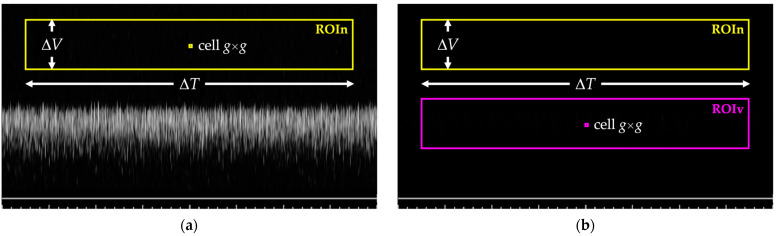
Example of ROIn and ROIv positioning on the spectral images to determine the maximum Doppler gain *G_max_* (a) and the minimum Doppler gain *G_min_* (b).
